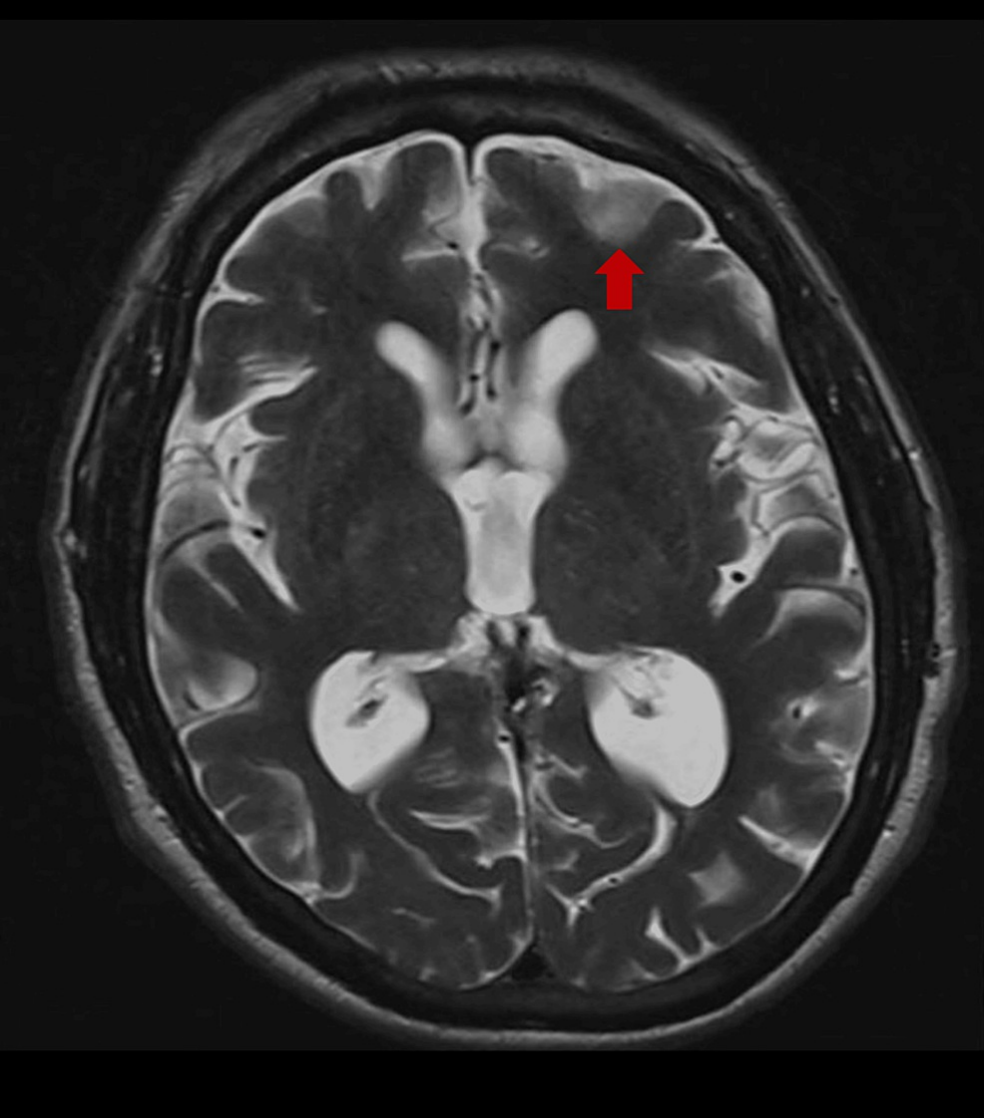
Enhancement of a deep left frontal lesion on T2-FLAIR imaging T2-FLAIR: T2-weighted fluid-attenuated inversion recovery
